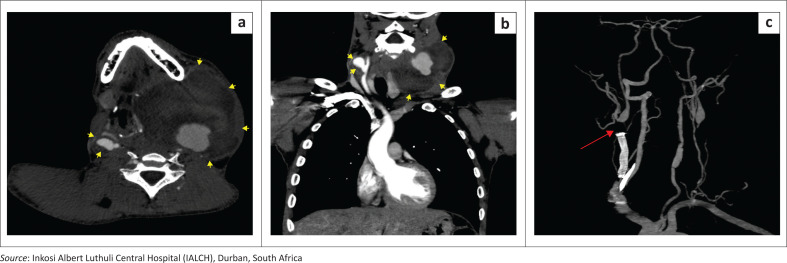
Clinical and imaging findings of a 40-year-old man on antiretroviral therapy presenting with a 3-month history of a progressively enlarging left-sided neck mass and obstructive airway symptoms. On clinical examination, a large left neck mass with overlying skin changes and blood oozing was noted. (a, b) CT demonstrated bilateral carotid aneurysmal disease (yellow arrowheads), including a ruptured left carotid pseudoaneurysm measuring 10.2 cm with a contained leak and a right carotid pseudoaneurysm measuring 2.8cm, both with circumferential intraluminal thrombi. The patient underwent open repair of the left pseudoaneurysm and right common carotid artery stenting. Re-exploration for soaked dressings revealed an intact left carotid patch with no active bleeding and evacuation of a 200-mL haematoma. (c) CTA showed occlusion of the left carotid stent and occlusion of the proximal right common carotid artery (red arrow).
